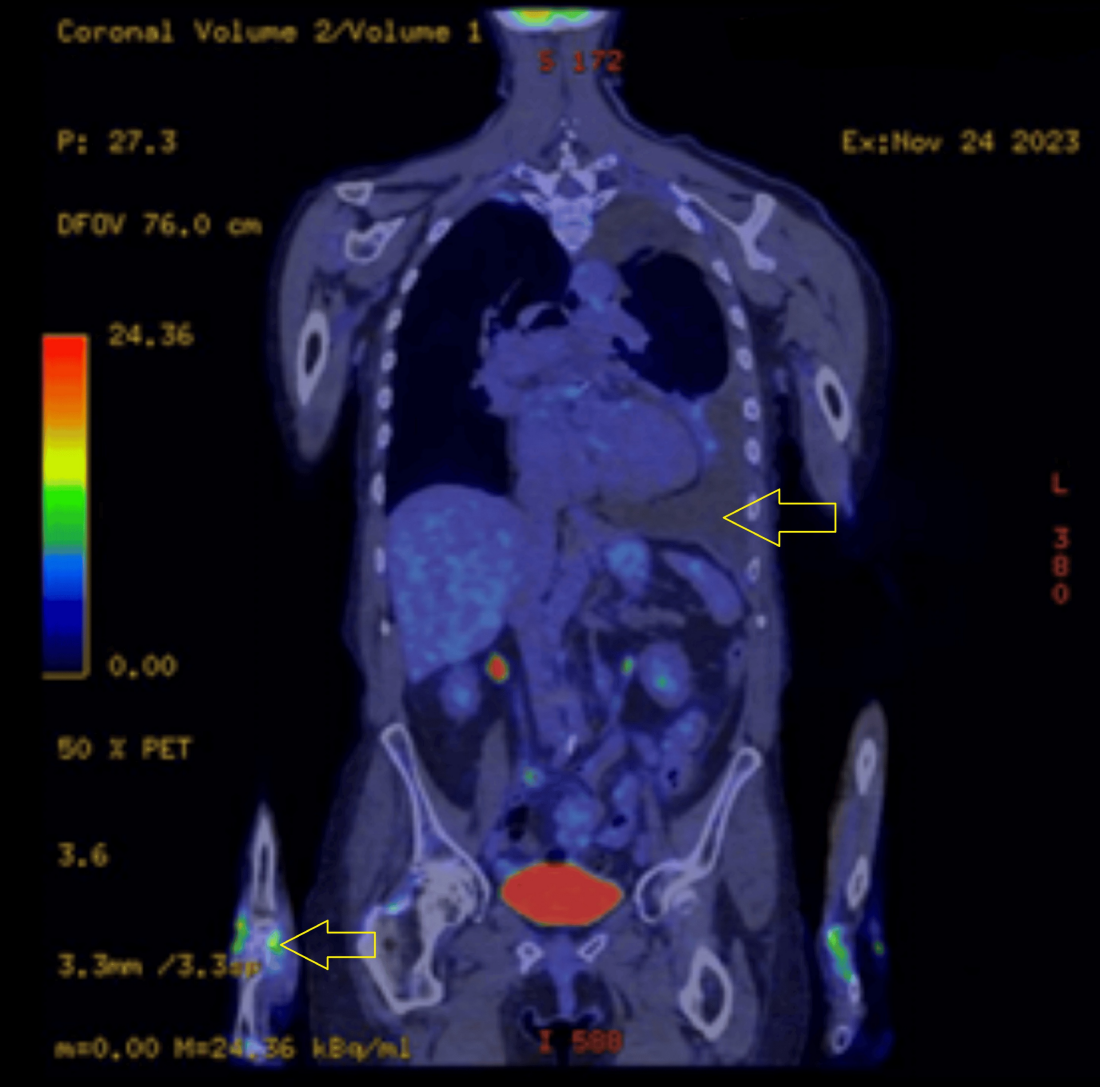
FDG PET-CT scan (coronal view) showing worsening left-sided effusion and persistent increased uptake in multiple joints.
